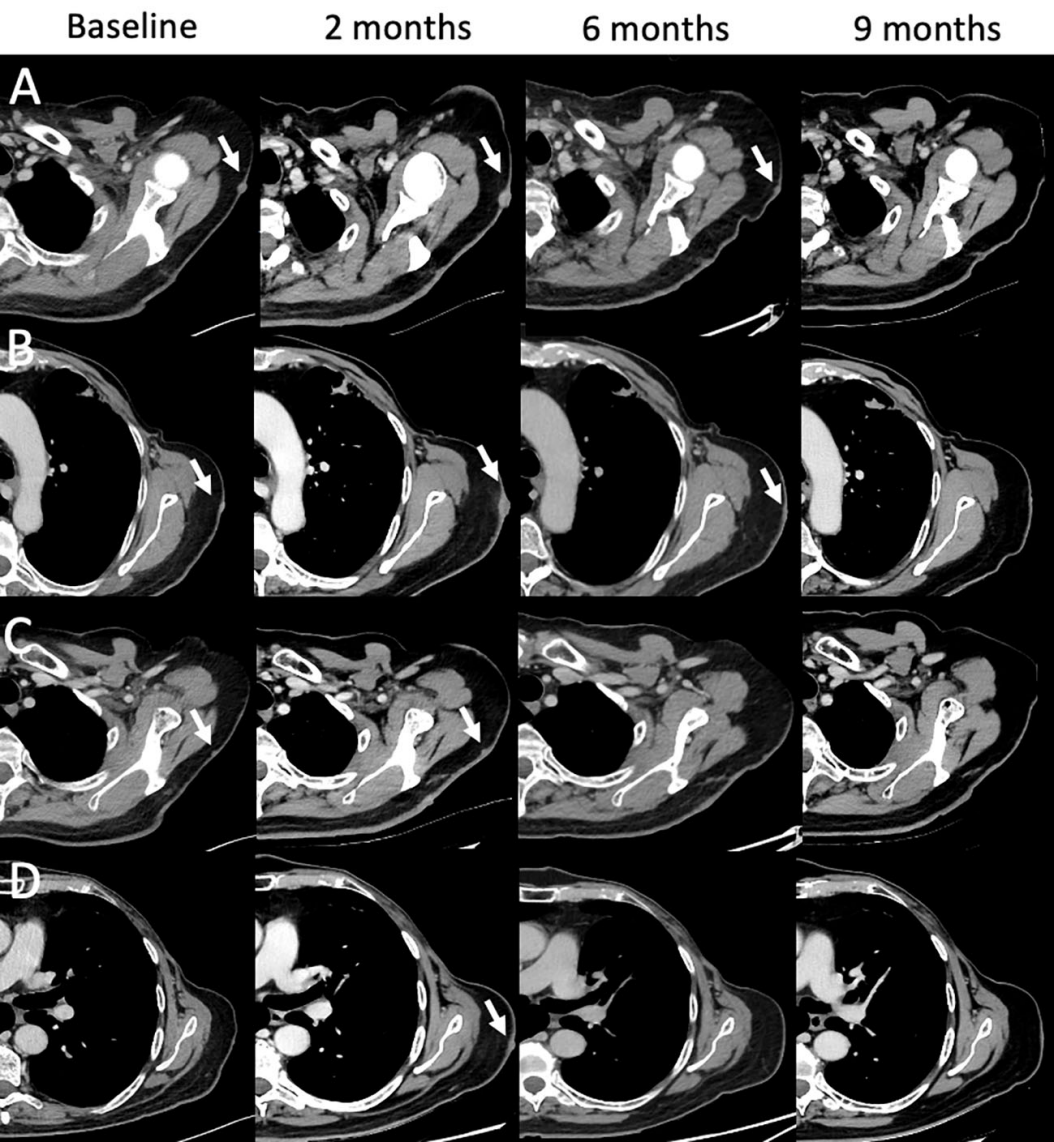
Mixed and complete responses following T-VEC therapy in a 66-year-old male with stage IIIB nodular multilesional melanoma in the left arm (baseline contrast-enhanced CT, arrows) previously treated with surgery and systemic immunotherapy with ipilimumab & nivolumab. Treatment with T-VEC was performed in up to 8 subcutaneous lesions per session. Following 3-4 treatment cycles, follow-up CTs were performed (at 2, 6, and 9 month). Representative examples of lesion evolution since baseline (A–D): Follow-up at 2 months showed mixed responses with progressive lesions [(A, B), arrows], stable lesions [(C), arrow] and new lesions [(D), arrow]. Follow-up at 4 months showed partial responses (A, B) and complete responses (C, D). Follow-up at 9 months showed complete response in all lesions (A–D). Treatment with T-VEC was continued.
